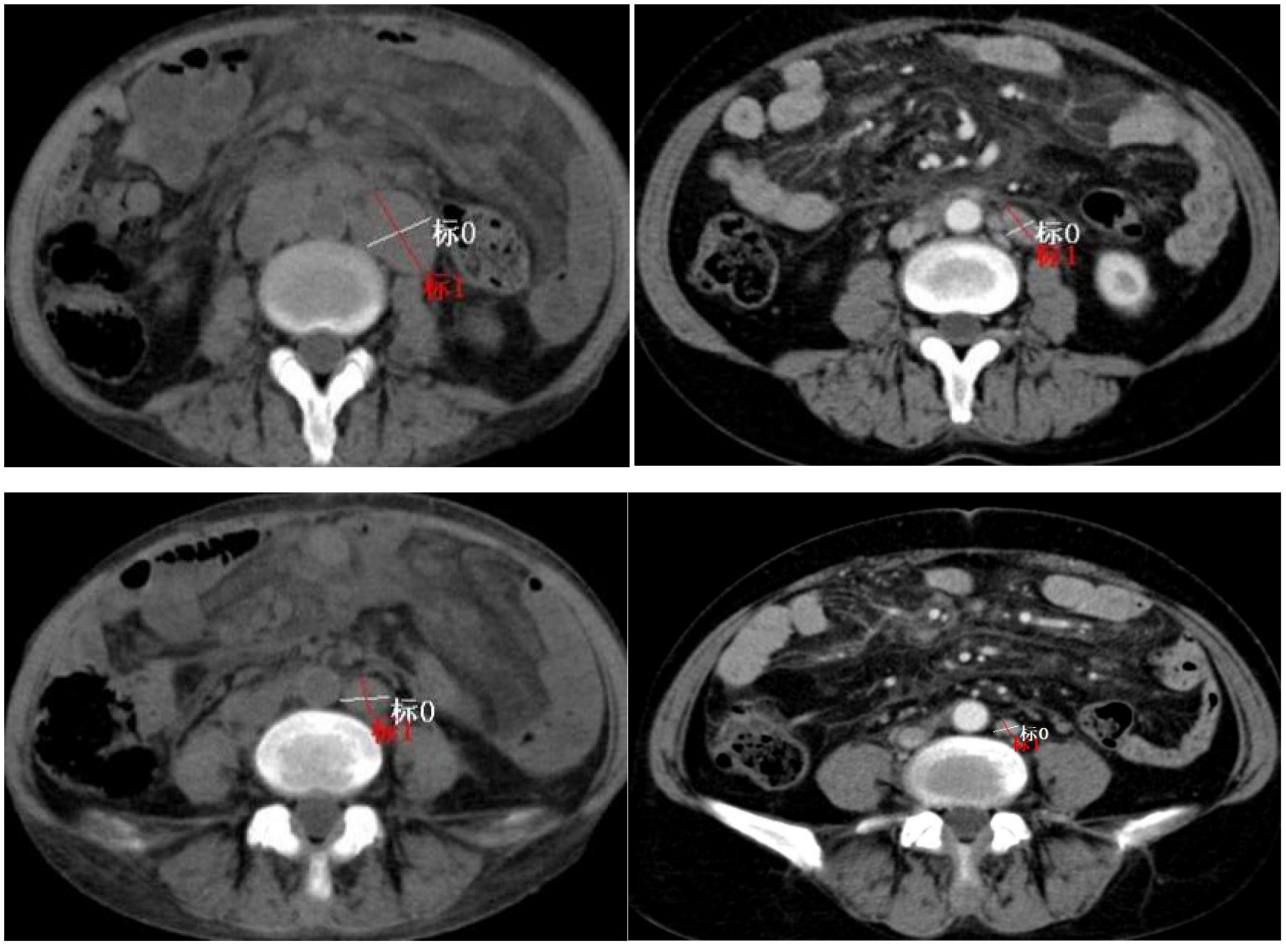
CT scan prior to initiation of PD-1 inhibitor treatment (12 Dec. 2024) (left), and six cycles after treatment initiation (3 Jun. 2025) (right).
